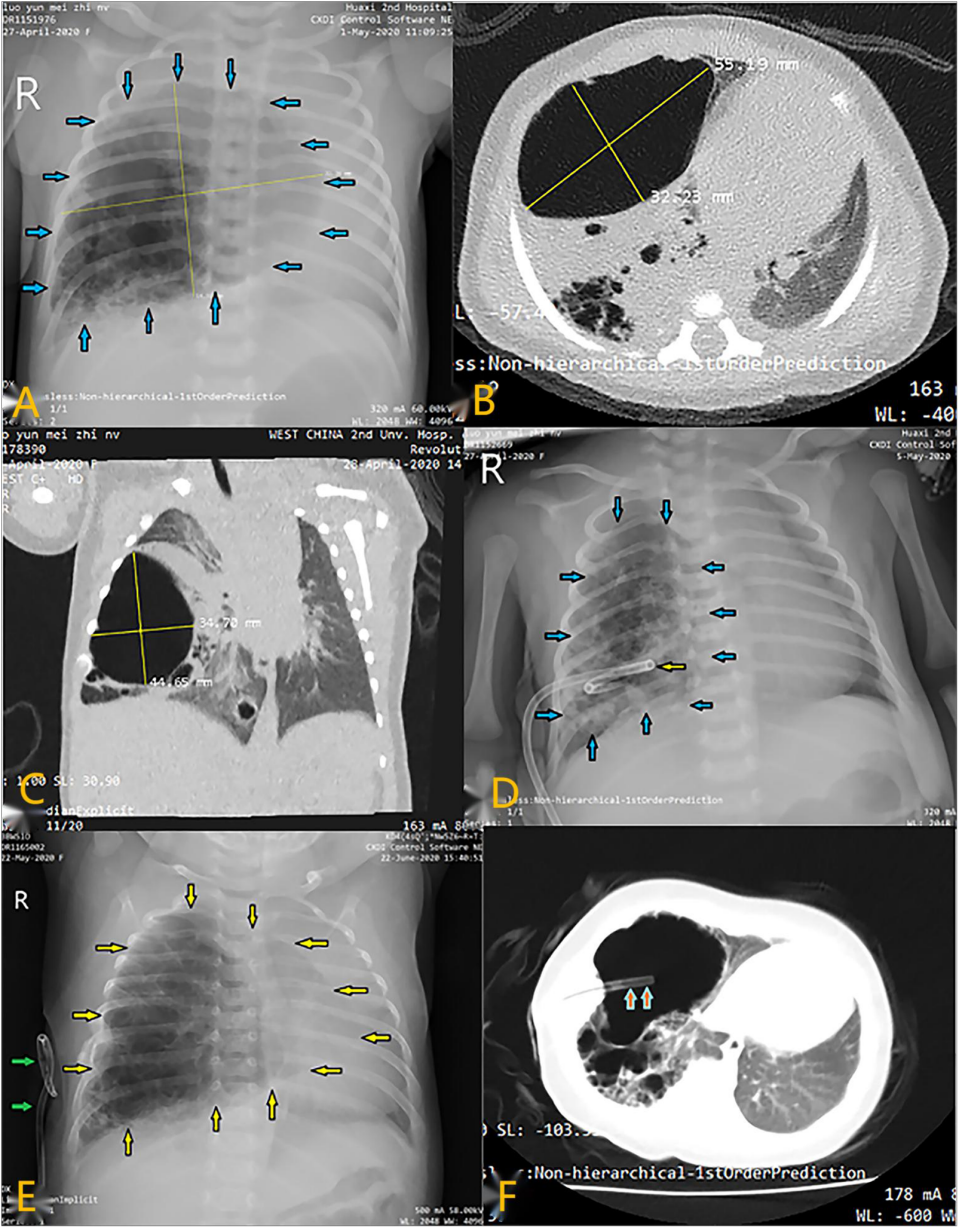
Radiological imaging of PTCD maneuvre in case 2. (A) Preoperative CT scan showed a large cystic lung lesion in the left lower lobe; (A–C) X-ray image showed a large cystic lesion (blue arrows) in the right thoracic cavity; CT scan indicated that the cystic lesion was so large that mediastinal displacement occurred; (D) Chest film demonstrated that a pigtail catheter (yellow arrow) was successfully placed in the lesional cyst; (E) Chest X-ray showed that the lesional cyst (yellow arrow) was restored to its original size when the catheter (green arrow) dislodged out of it; (F) CT scan indicated suction catheter (orange arrow) was successfully placed into the large cyst along the existing fistula in the thoracic wall.
